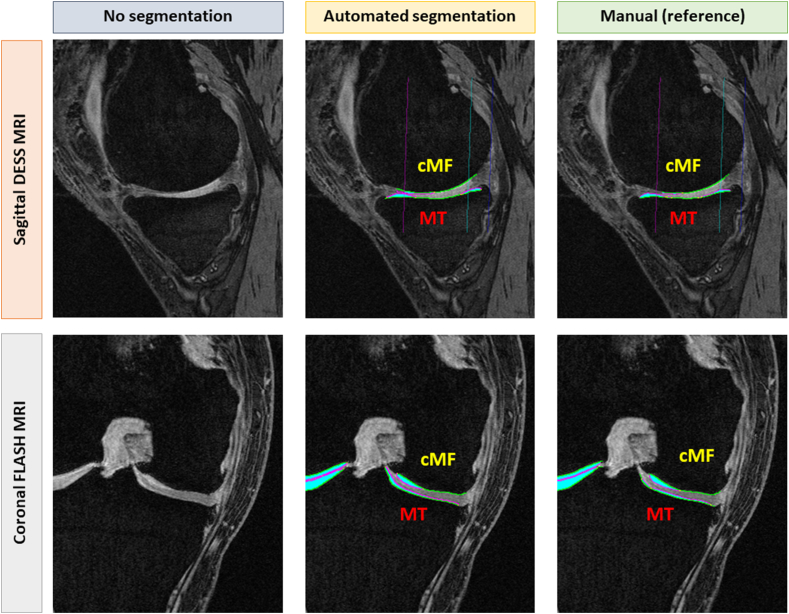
Sagittal DESS MRI (top row) and coronal FLASH (bottom row), without cartilage segmentation (left column), with automated AI-based cartilage segmentation (middle column), and with manual (reference) cartilage segmentation of the medial femoro-tibial compartment (MFTC). MT ​= ​medial tibia; cMF ​= ​weight-bearing (central) medial femur.
